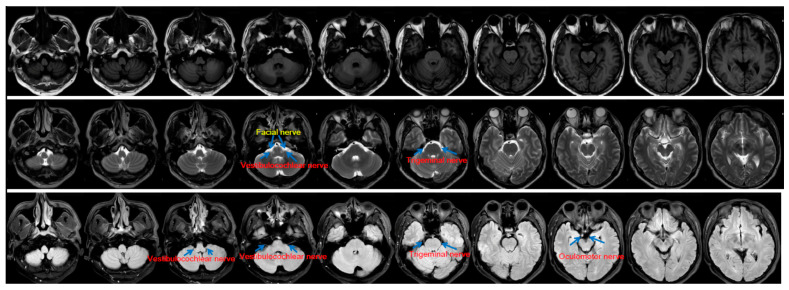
Cranial nerves on clinical routine MRI. T1, T2, and Flair MRI images with a low spatial resolution (5-mm slice thickness in the axial plane with a 1-mm gap between slices) acquired by using a 3T MRI scanner are shown in the first, second, and third rows. Note that fewer cranial nerves can be seen in this figure than in Figure 1.
